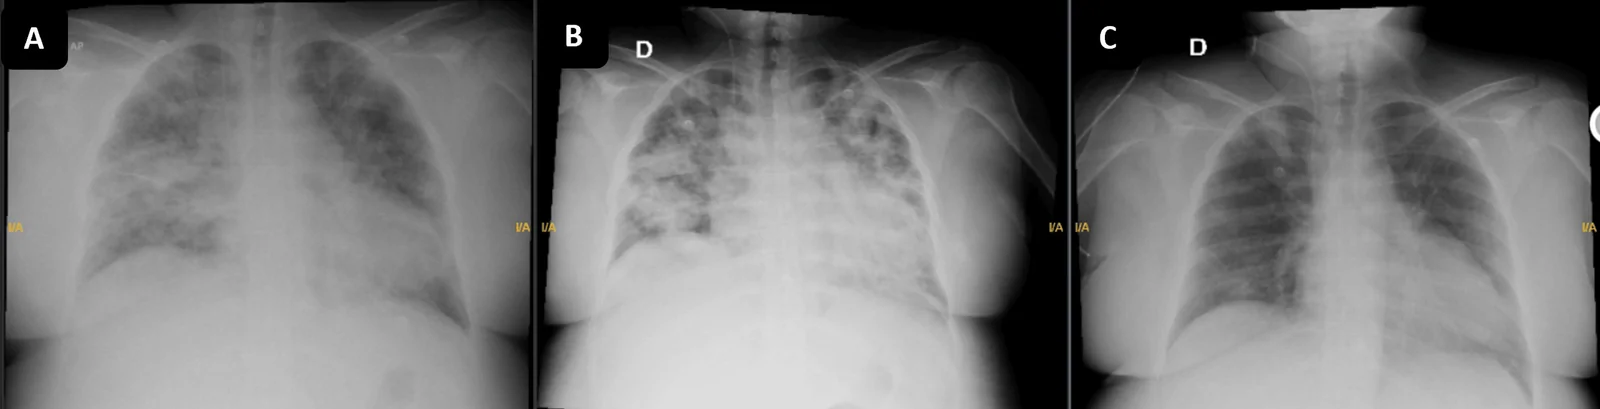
Serial chest radiographs during pulmonary DS. (A) Chest radiograph obtained 48 hours after ATRA initiation, showing diffuse bilateral alveolar-interstitial opacities, predominantly in the perihilar and basal lung fields, consistent with severe pulmonary involvement in the setting of acute hypoxemic respiratory deterioration. (B) Follow-up radiograph after 48 hours of HFNC support, showing persistent bilateral alveolar-interstitial opacities, which prompted escalation to pulse methylprednisolone therapy. (C) Follow-up radiograph obtained after three days of pulse methylprednisolone therapy and continued HFNC support, showing marked radiologic improvement with significant resolution of the bilateral pulmonary opacities. ATRA, all-trans retinoic acid; DS, differentiation syndrome; HFNC, high-flow nasal cannula
